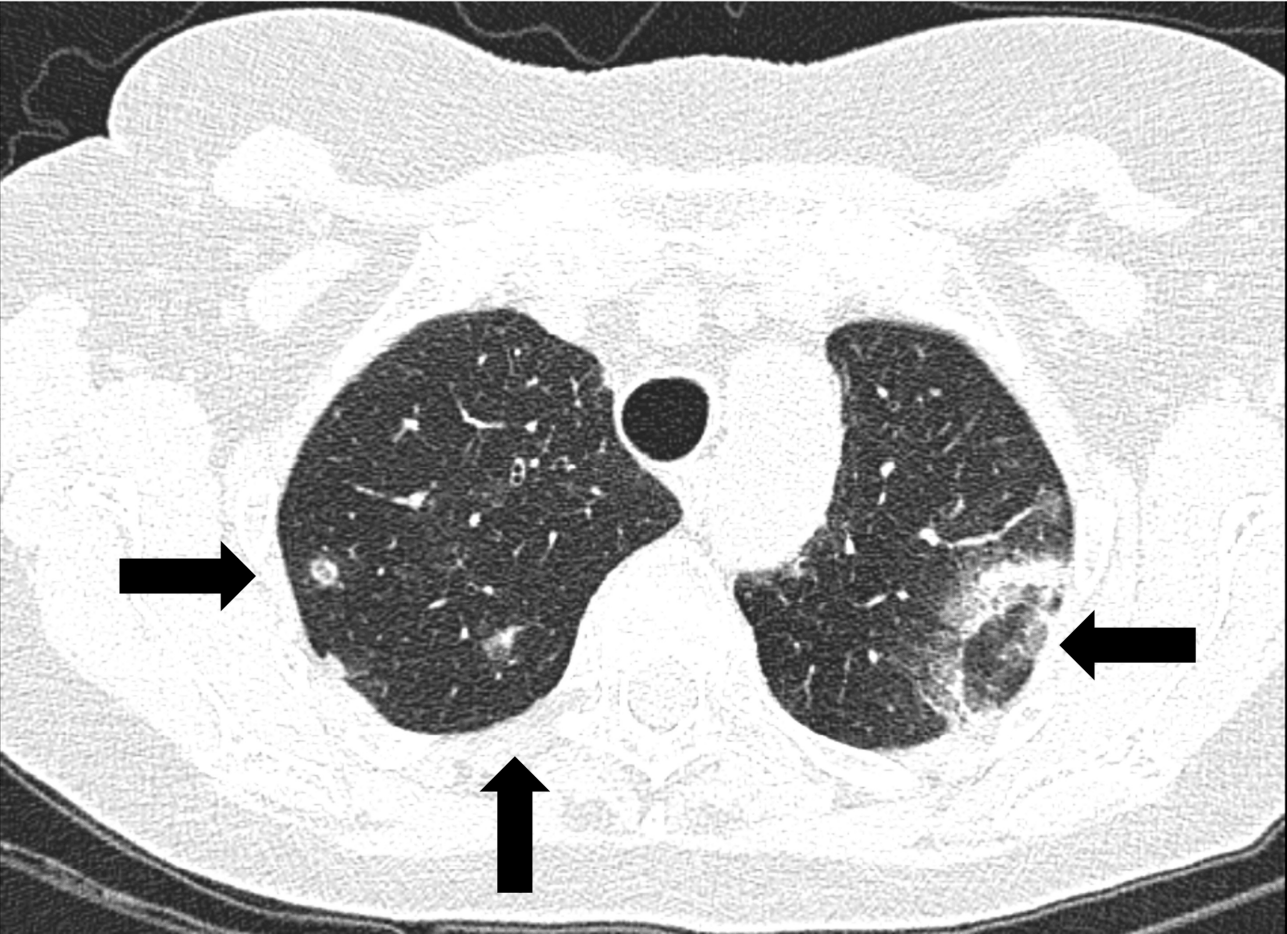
Thoracic CT scan revealing new areas of parenchymal consolidation localized to both pulmonary apexes (new location; second relapse). New areas of consolidation at the pulmonary apexes bilaterally (black arrows).
